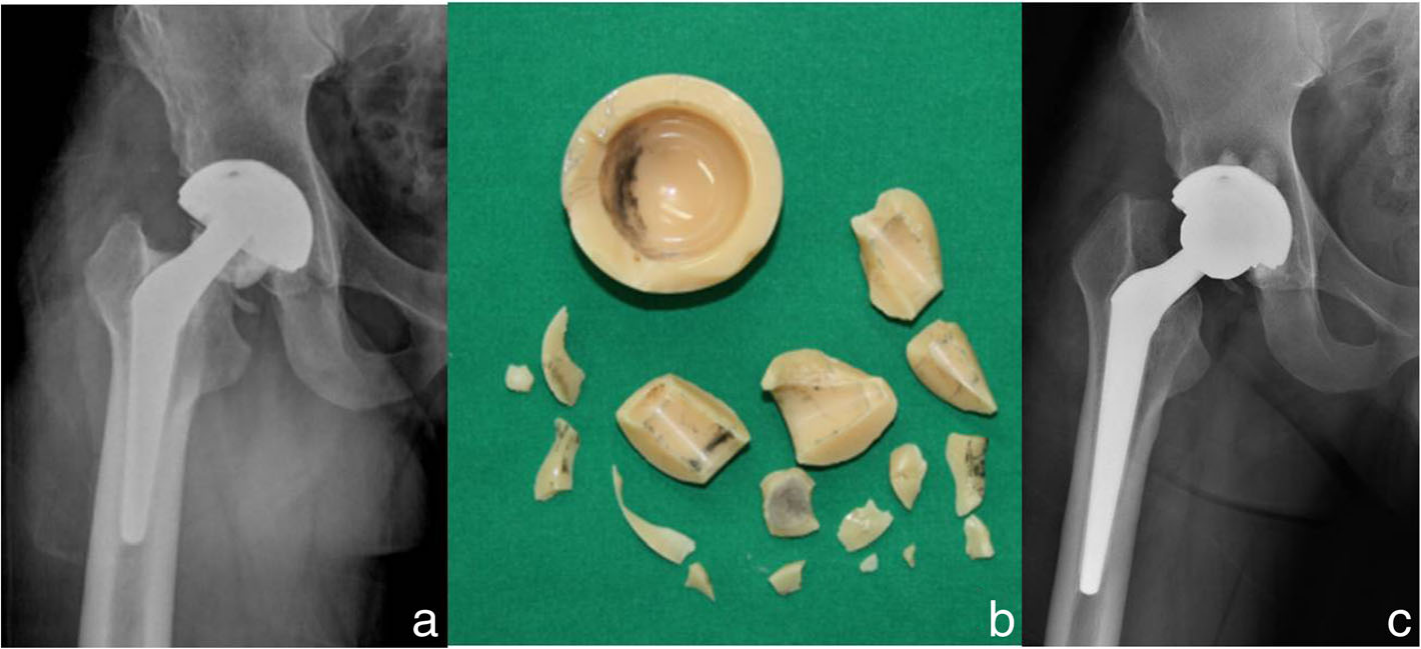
A 34-year-old male patient with right total hip arthroplasty using CoC articulation (Forte) (a) Radiograph with fractured ceramic head and liner (b) The fractured ceramic head and liner (c) Radiographs after revision surgery changing the ceramic liner and fractured head to metasul liner and metal head after cementing
